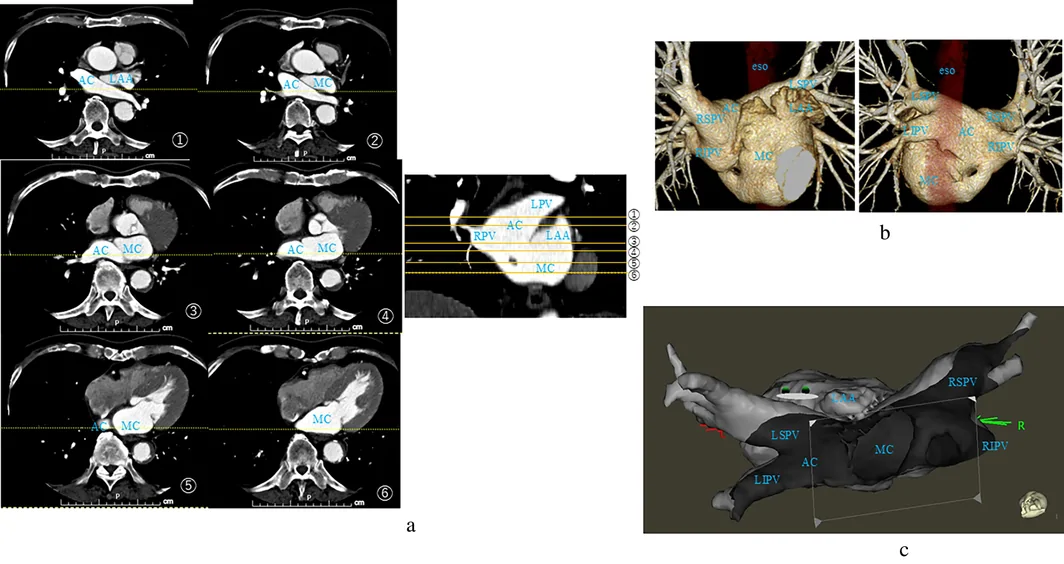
Multi‐detector computed tomography (MDCT) findings. (a) Coronal MDCT image of the LA (right) with corresponding numbered axial sections (left). (b) Three‐dimensional reconstruction showing all pulmonary veins (PVs) draining into the AC. (c) Three‐dimensional reconstruction with a superior inner view demonstrates that the LA is partitioned by a membrane containing two fenestrations. AC, accessory chamber; LA, left atrium; LAA, left atrial appendage; LS, left superior; LI, left inferior; MC, main chamber; PV, pulmonary vein; RS, right superior; RI, right inferior.
